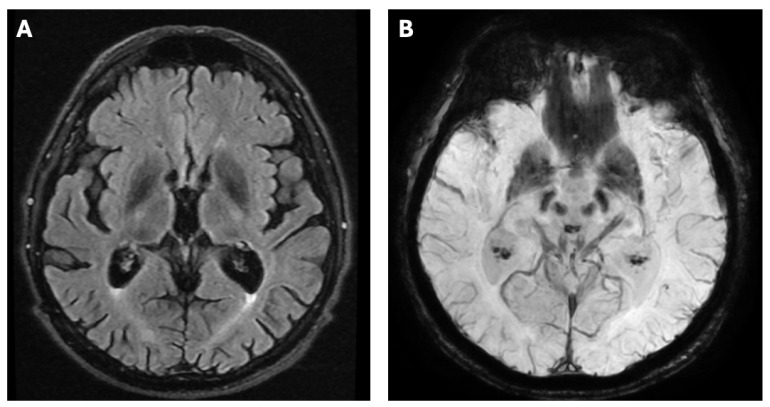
Brain MRI of the patient showing reduced signal intensity symmetrically in the putamen, globus pallidus, and caudate nuclei on the T2-weighted sequence (A); reduced signal intensity symmetrically involving the putamen, globus pallidus, red nuclei, and substantia nigra on the SWI sequence (B).
